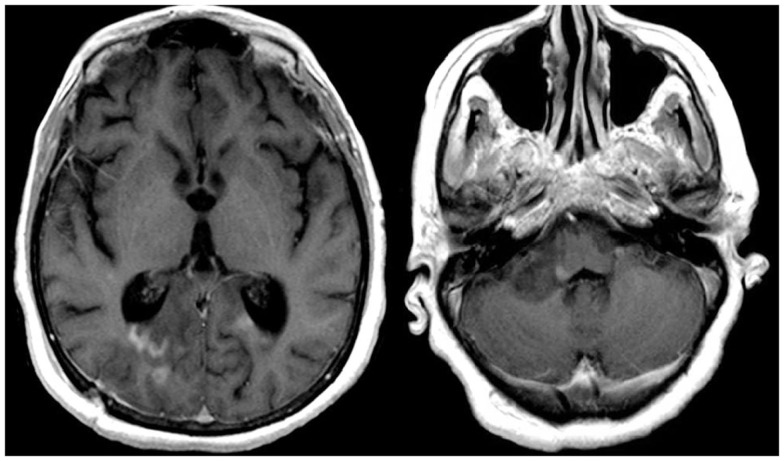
T1W-MRI with contrast shows lesions located in the splenium, pons, and cerebellum with minimal contrast enhancement.
